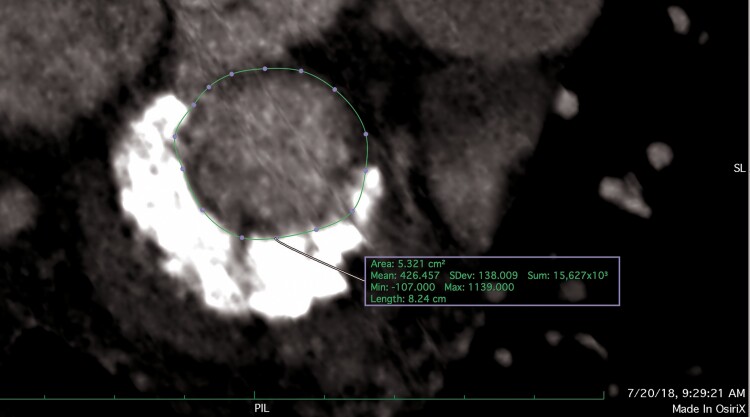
Assessment of mitral valve area for transcatheter mitral valve replacement on multiplanar cardiac-gated computed tomography.
